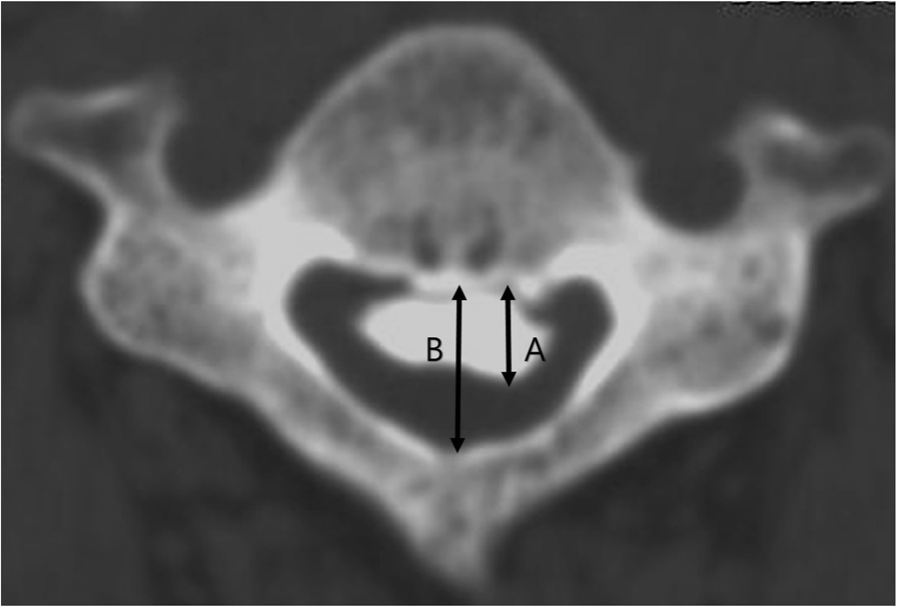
Measurement of the space available for the spinal cord (SAC). SAC was calculated on the basis of axial computed tomography. *B* = developmental canal diameter. *B-A* = space available for the spinal cord (SAC)
